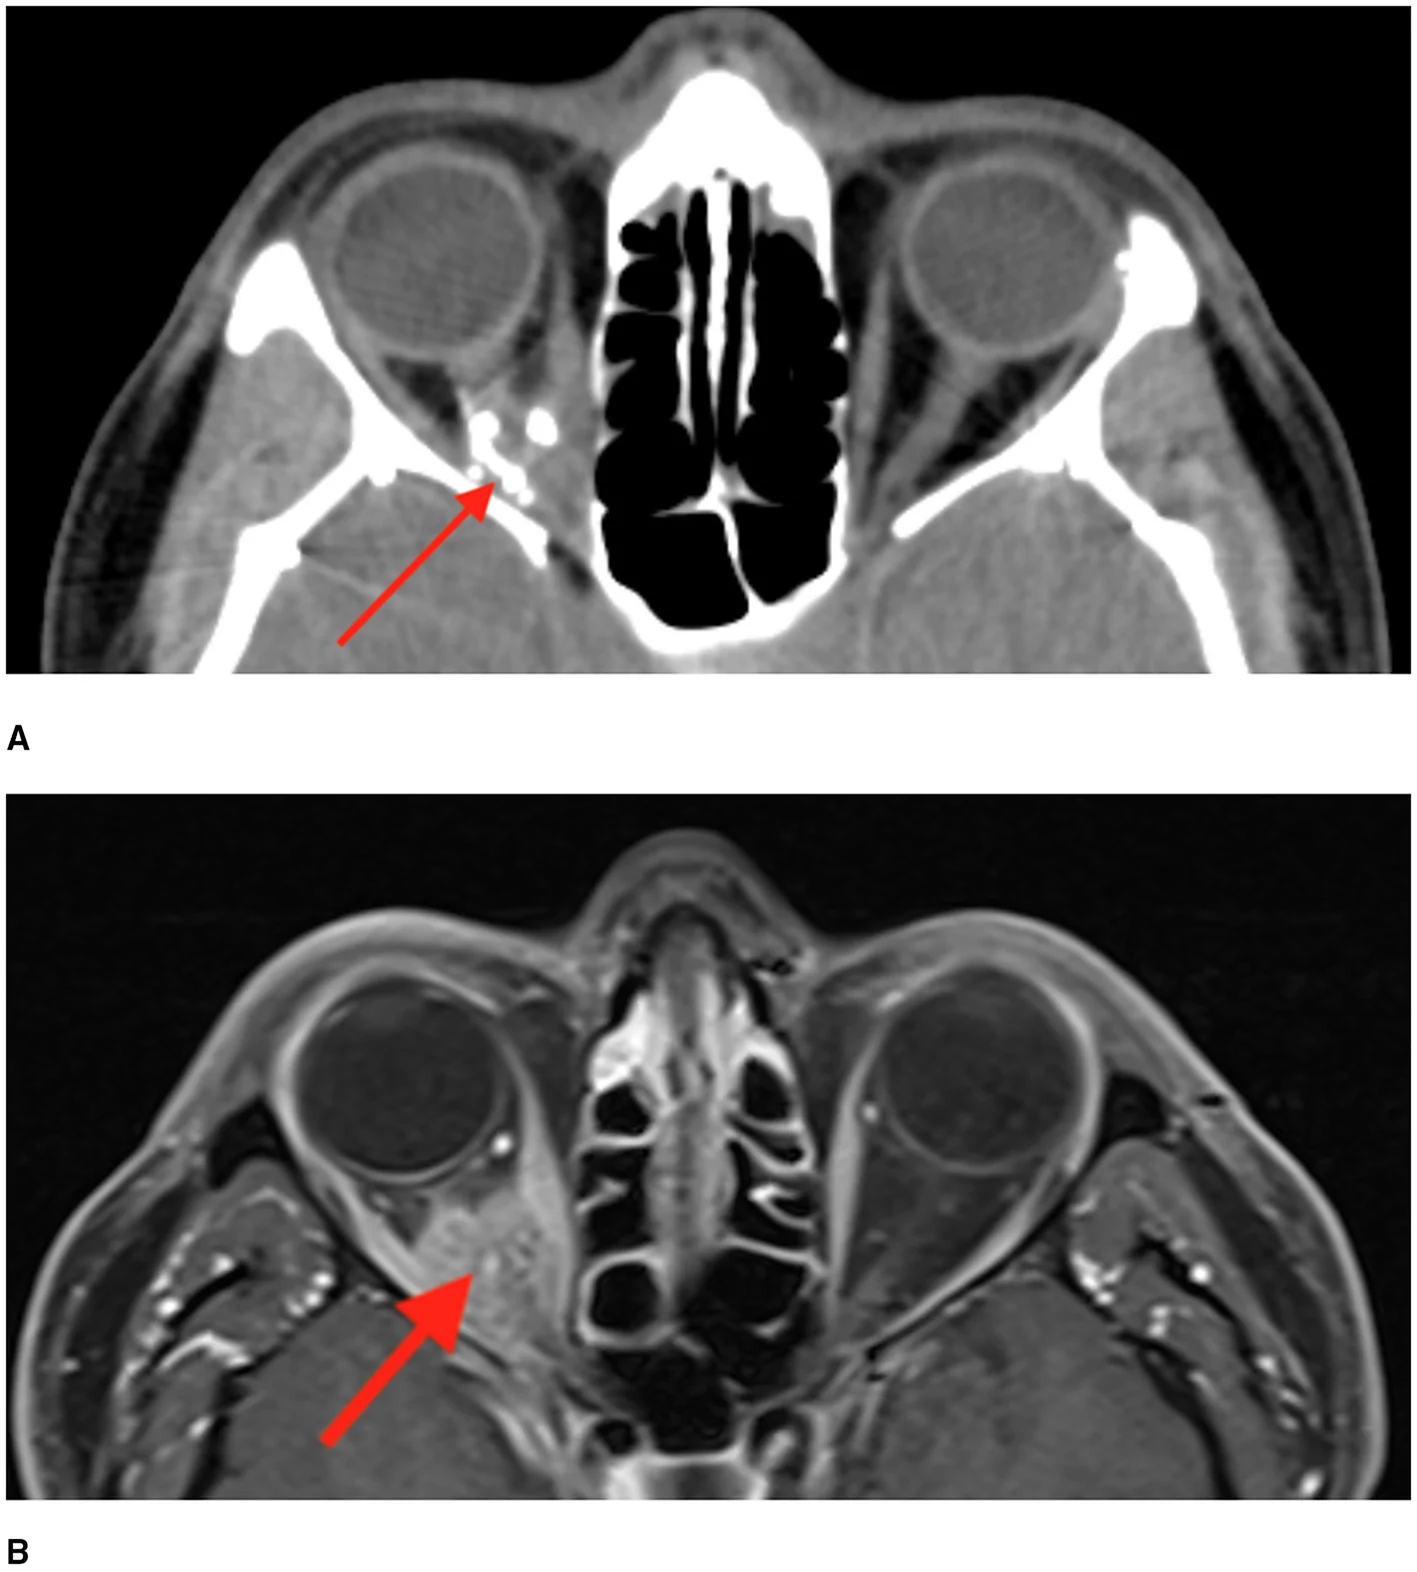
(A) CT Orbits without contrast from case 2 obtained 1 year after embolization. Arrow points to suspected embolization material. (B) MRI orbits axial T1 post contrast image with fat suppression obtained approximately 1 year after CT scan from 3A. Arrow Points to enhancement compatible with granulomatous reaction to embolization material.
